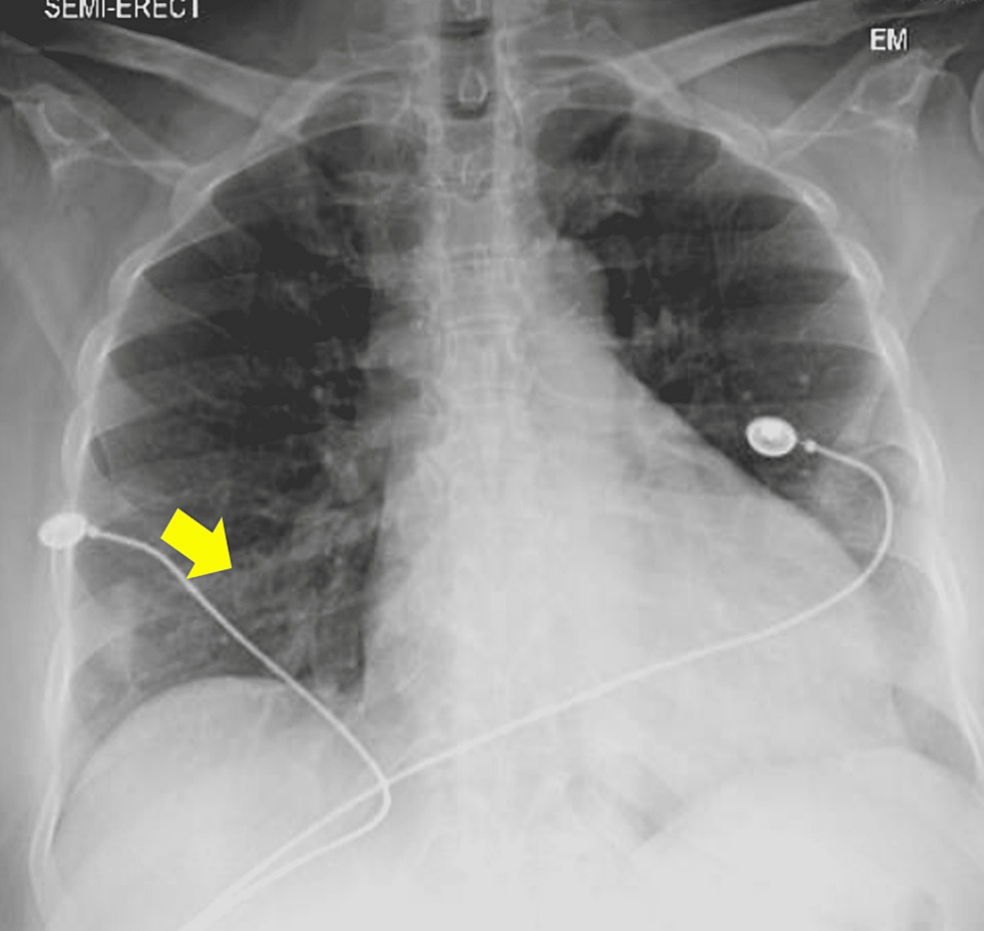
Chest X-ray showing nodular densities in the right lower lung field (yellow arrow).
